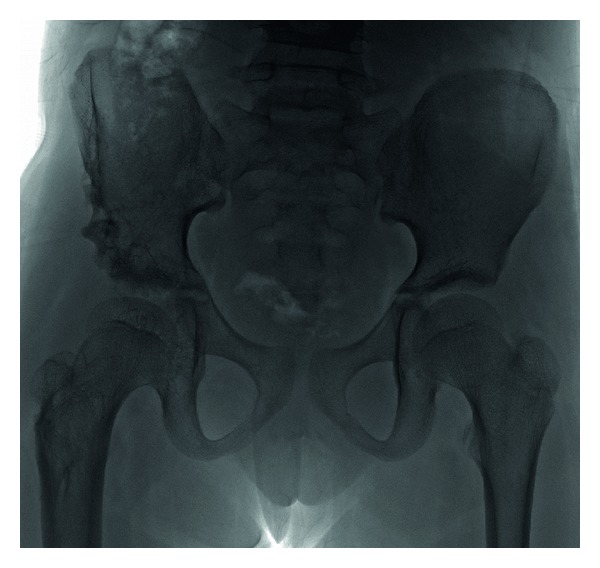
Control plain radiograph, five years after surgery.
